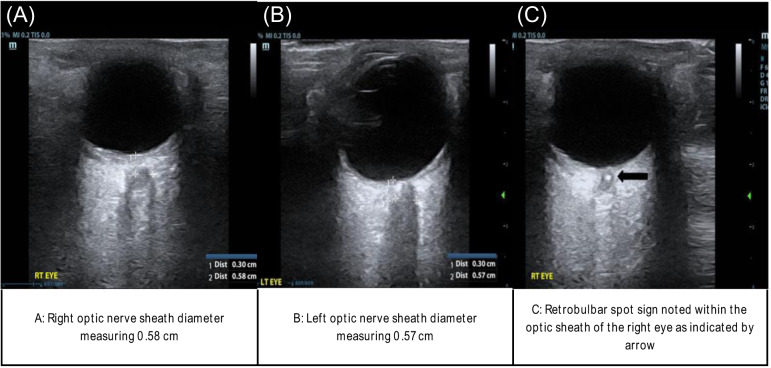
Point-of-care-ultrasound findings demonstrating normal right- (A) and left-sided (B) optic nerve sheath diameters, as well as an acute thrombus in the central retinal artery called the retrobulbar spot sign (C). *cm*, centimeter.
